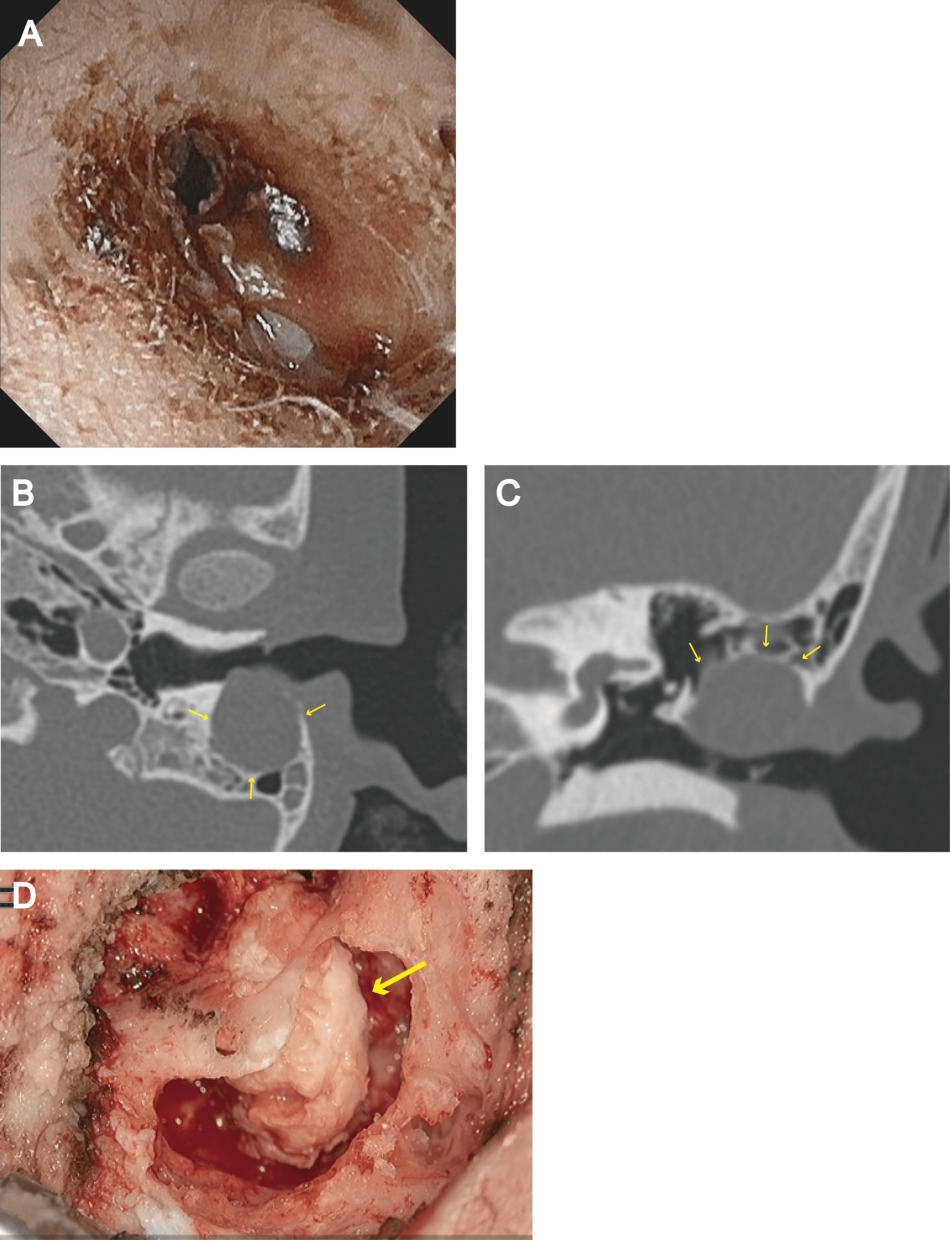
Preoperative and intraoperative findings. (A) Preoperative otoscopic findings. Tightly compacted granulation tissue almost filled the external auditory canal. Preoperative axial (B) and coronal (C) computed tomography (CT) images of the temporal bone. A round mass with a smooth, well-defined margin (yellow arrows) compressed and destroyed the posterior wall of the external auditory canal. The mass did not involve the mastoid tegmen, sigmoid sinus, or tympanic membrane. (D) Intraoperative findings. After removing the mastoid cortex, a cholesteatoma (yellow arrow) was found in the mastoid cavity.
